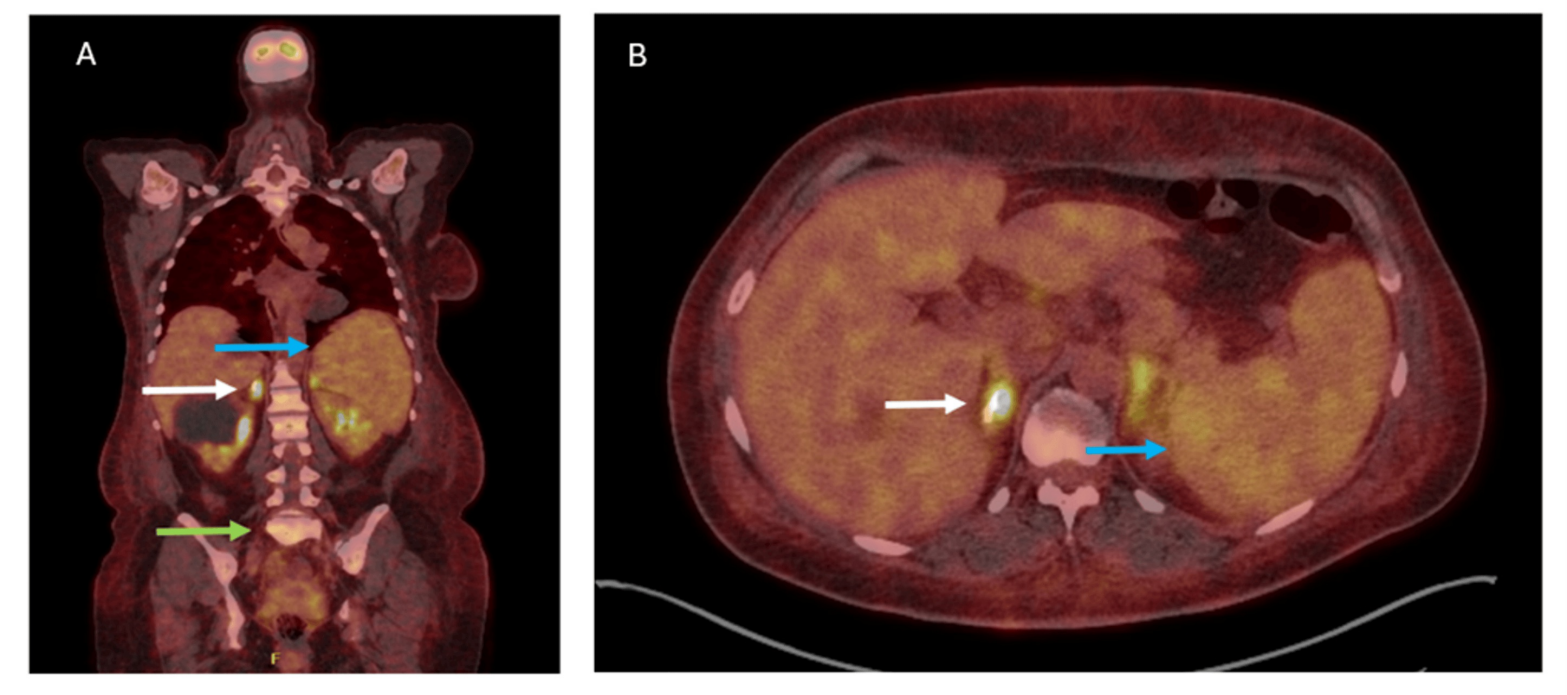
PET-CT findings in our patient Increased 18F-fluorodeoxyglucose uptake in the adrenal glands (white arrows), bone marrow (green arrow), and spleen (blue arrows). (A) Coronal fused view and (B) axial fused view.
